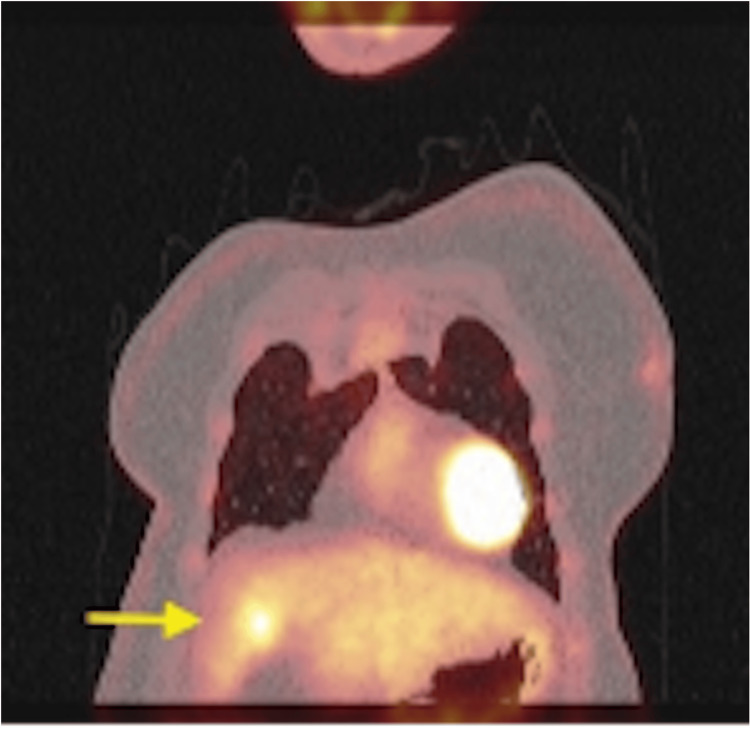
Coronal fused 18F-fluorodeoxyglucose positron emission tomography/computed tomography (18F-FDG PET/CT) image confirming isolated FDG uptake in the hepatic lesion (yellow arrow), with no evidence of extrahepatic disease.
